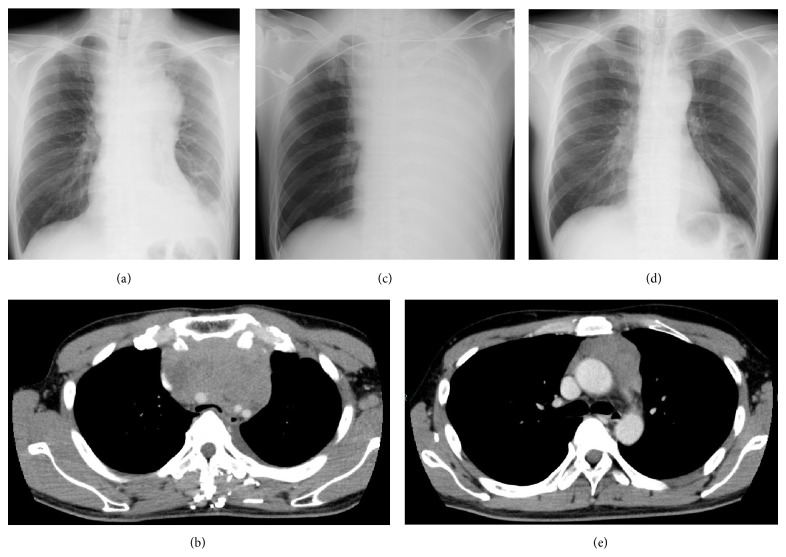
A CXR performed on admission shows a widened mediastinum (a). A contrast enhanced CT on admission shows a mediastinal tumor compressing trachea (b). A CXR performed after intubation on day 2 shows atelectasis of the left lung and a venous cannula inserted for ECMO (c). A CXR performed after one course of consolidation chemotherapy on day 71 shows disappearance of atelectasis and shrinkage of the mediastinal tumor (d). A contrast enhanced CT on hospital day 87 shows partial remission of the mediastinal tumor (e).
